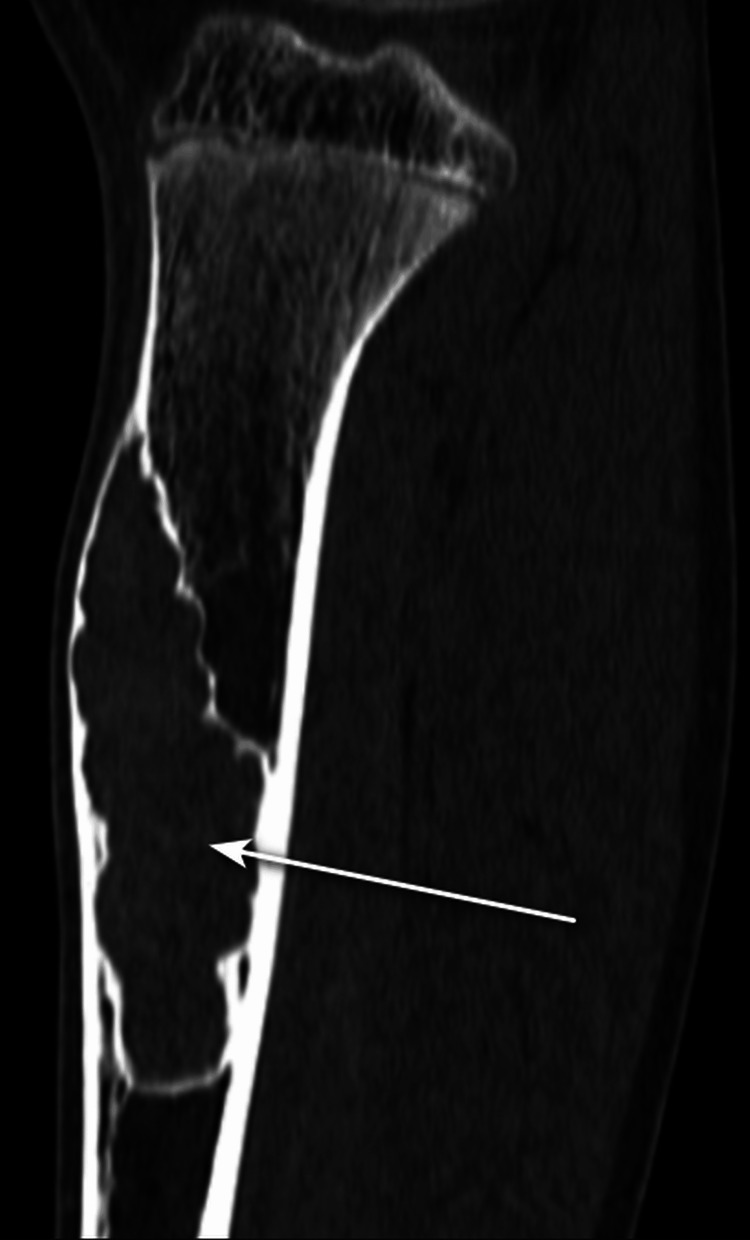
Sagittal CT section of the tibia in bone window showing expansile lytic lesion (white arrow). CT: computed tomography
